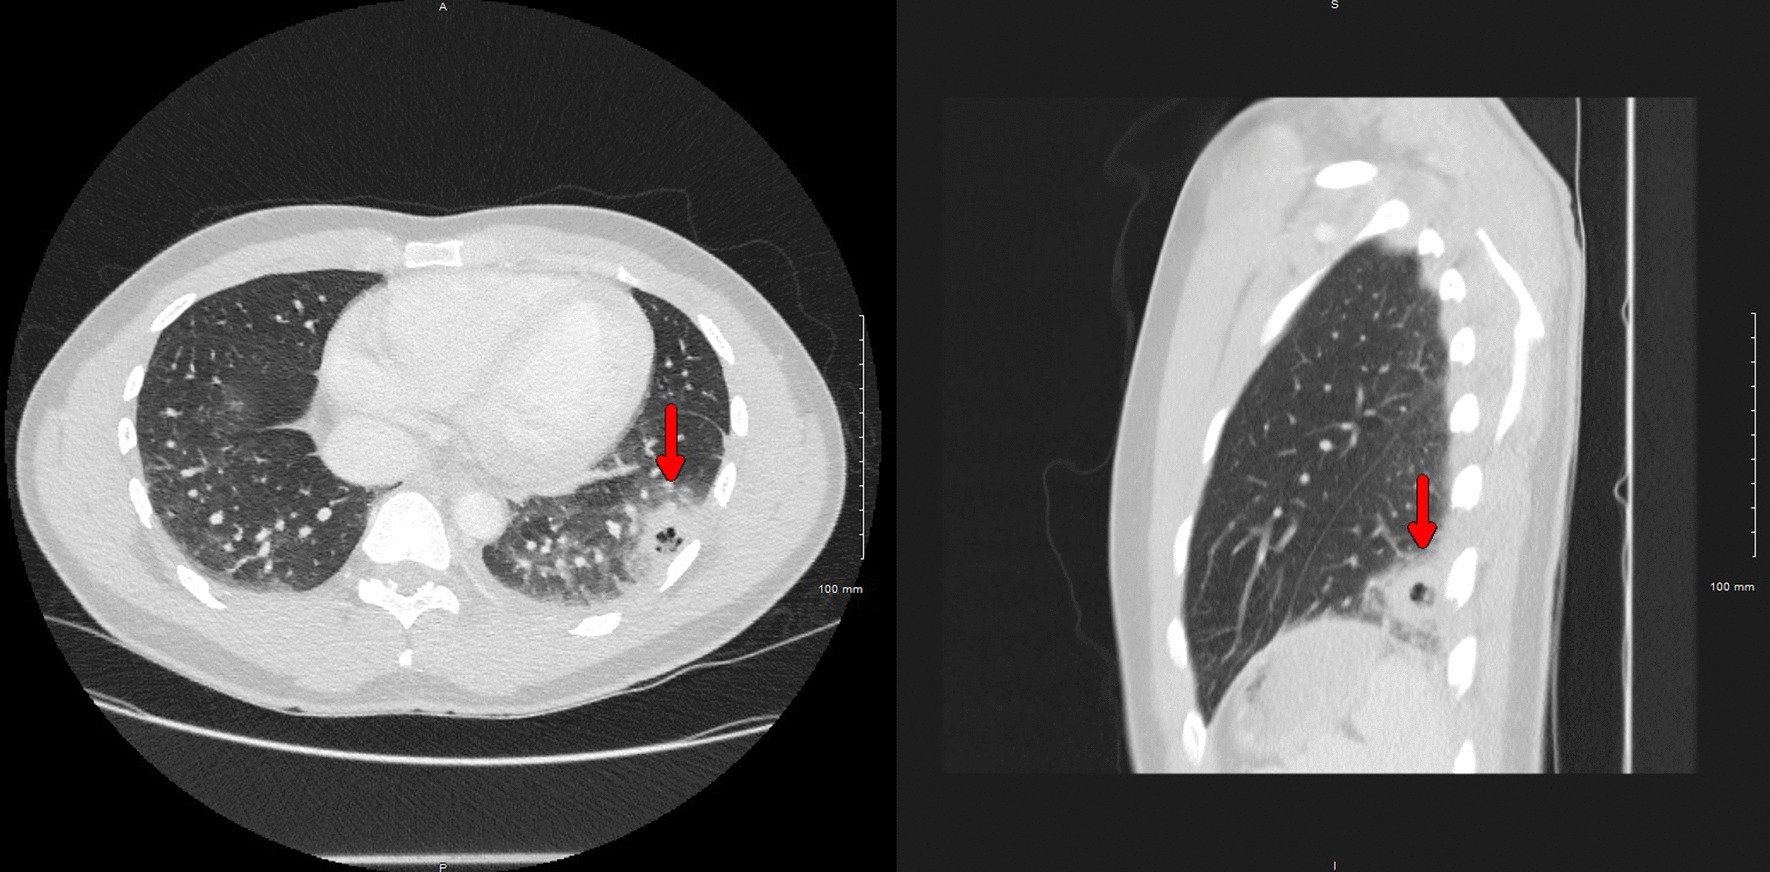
Chest CT showing a left lower lobe cavitating mass measuring up to 3 cm (red arrows) and peripheral ground-glass opacities within the lung bases bilaterally (right: axial plane; left: sagittal plane)
